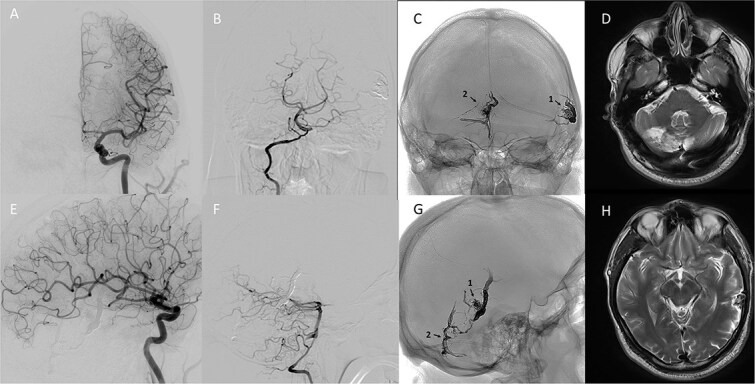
3 months follow up. A, E - DSA from the left ICA showing absence of cerebral AVM contrast, frontal (A) and lateral (E) views; B, F - DSA from the right vertebral artery showing absence of cerebral AVM contrast, frontal (B) and lateral (F) views; C, G - craniograms showing embolic agent’s carsts of multiple cerebral AVMs, frontal (C) and lateral (G) views; D - T2 weighted brain MRI in axial view showing formed postischemic cerebellar cyst; H - T2 weighted brain MRI in axial view showing regression of edema around a total embolized AVM of the left temporal lobe. Arrows: 1 - Squid (Balt) carst in the left temporal lobe AVM; 2 - Squid (Balt) carst in the cerebellar AVM.
